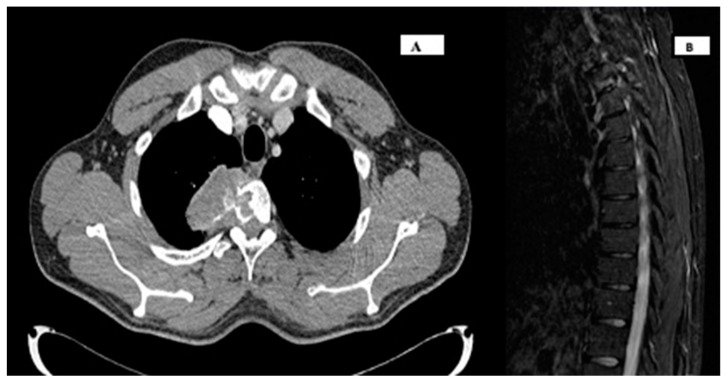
A/B. Re-evaluation studies after neoadjuvant treatment (January 2023). (A) Thoracic CT scan showing response to treatment, with a significant decrease in the size of the right posterior costovertebral mediastinal mass, with diameters currently approximately 3.5 × 5.9 × 4.3 cm (AP × T × CC) and similar invasion/affectation of the right side of the T2 and T3 vertebrae, respecting the contiguous T1 and T4 vertebrae. (B) MRI of the dorsal spine also showing a marked decrease in the soft tissue mass associated with lytic lesion in the right lateral mass of the T3 vertebral body and a decrease in the dimensions of the spinal canal on the right side without obliteration of the exit foramina of the T2 and T3 roots.
